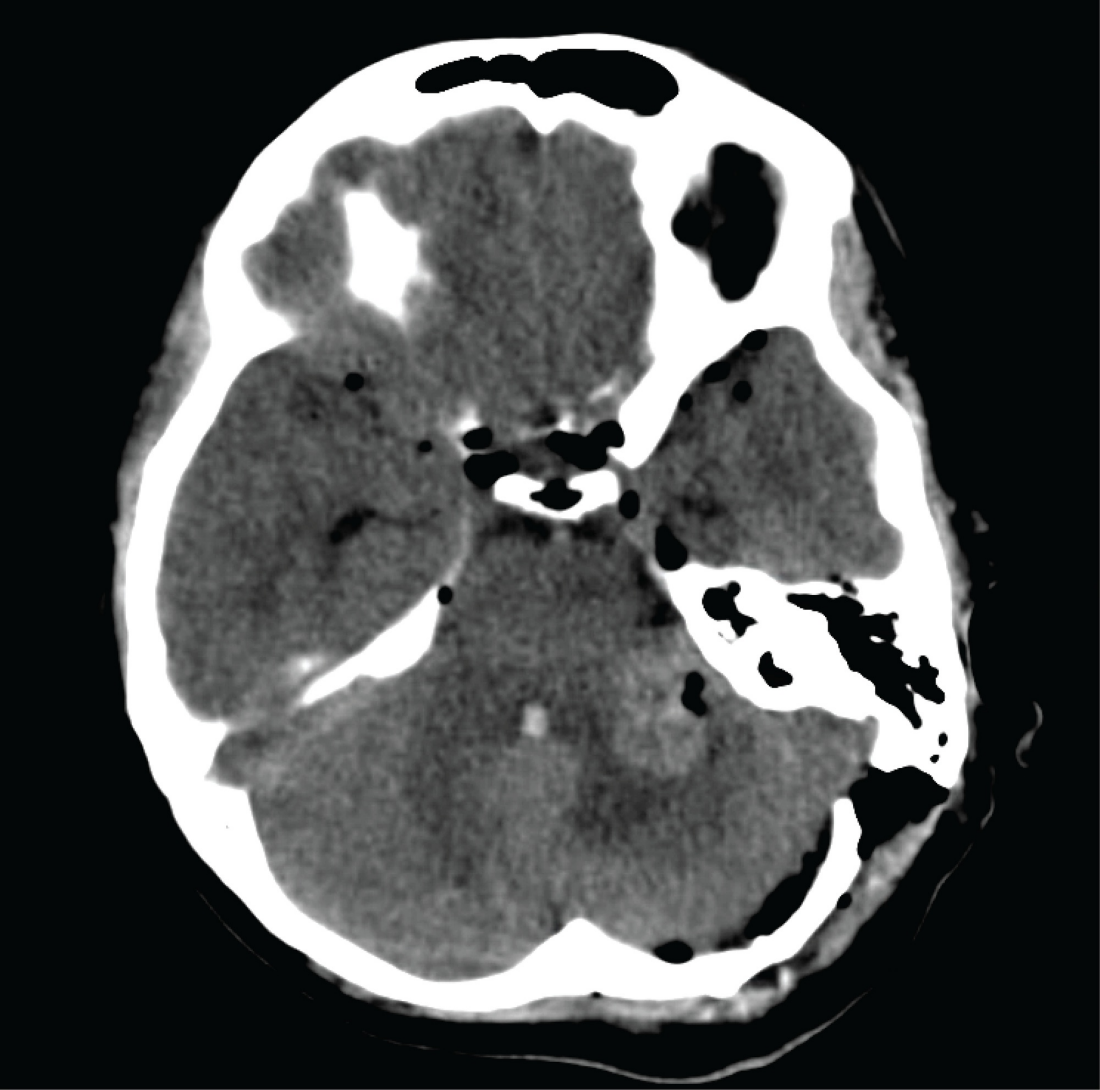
Postoperative brain CT of the patient The left retromastoid entry point is visualized by the defect in the temporal bone. Subtotal resection of the tumor shows decompression of the CPA. CT: computed tomography; CPA: cerebellopontine angle
